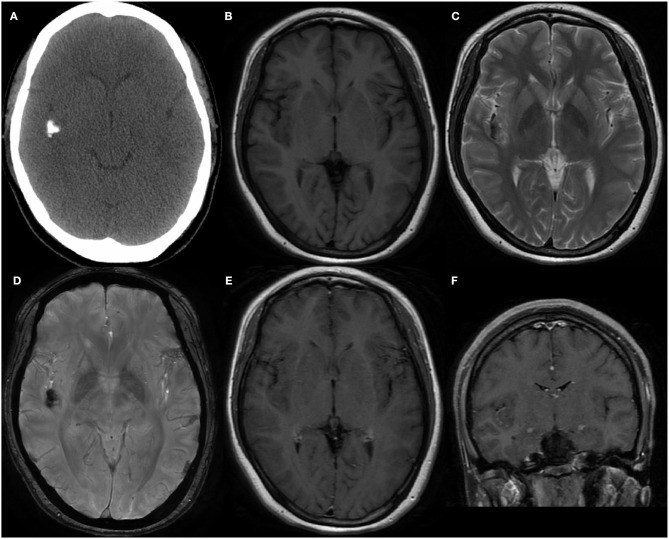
(A) The Computed tomography demonstrated the calcification in right Sylvian fissure and posterior part of insula. (B,C) MRI scans revealed a lesion without dural attachment located in right insular lobe which was mainly hypointense on both T1-weighted and T2-weighted. (D) Lesion showed hypointense on susceptibility weighted imaging. Enhanced MRI showed no obvious enhancement after gadolinium administration in axial (E) and coronal (F) position.
